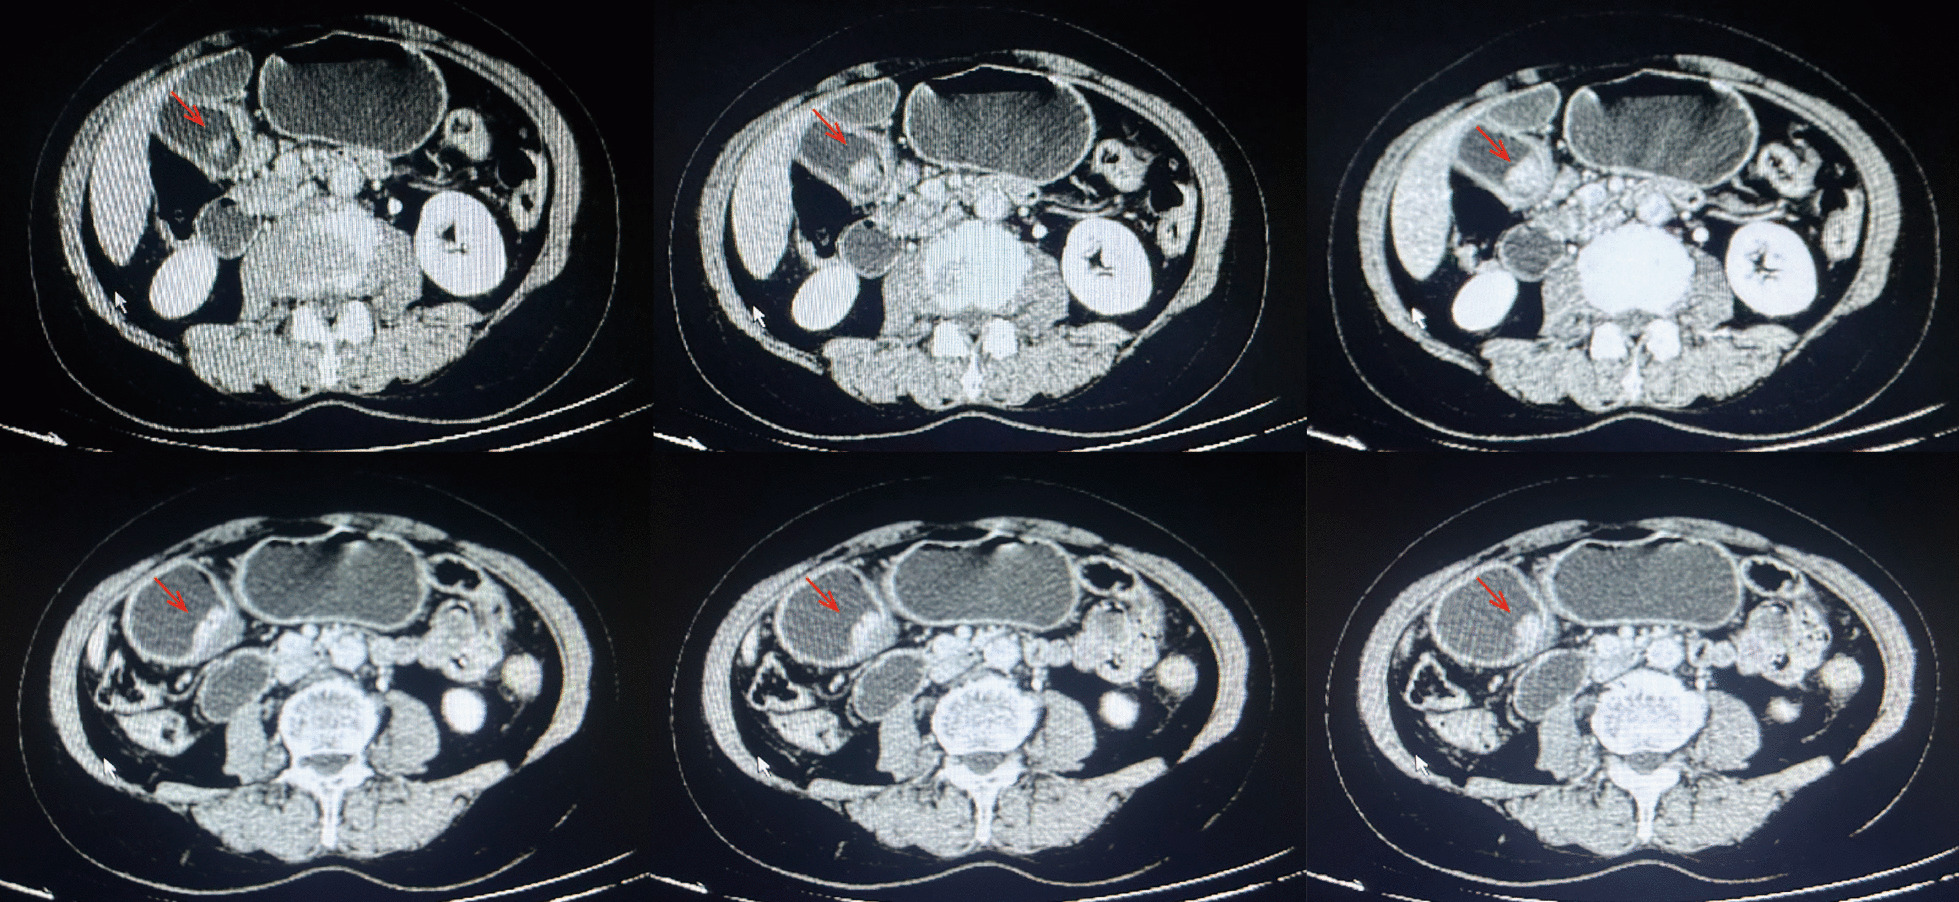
Gastric Computed tomography revealed mucosal thickening and ulceration in the gastric antrum, with para-antrum lymph node metastasis(arrows)
